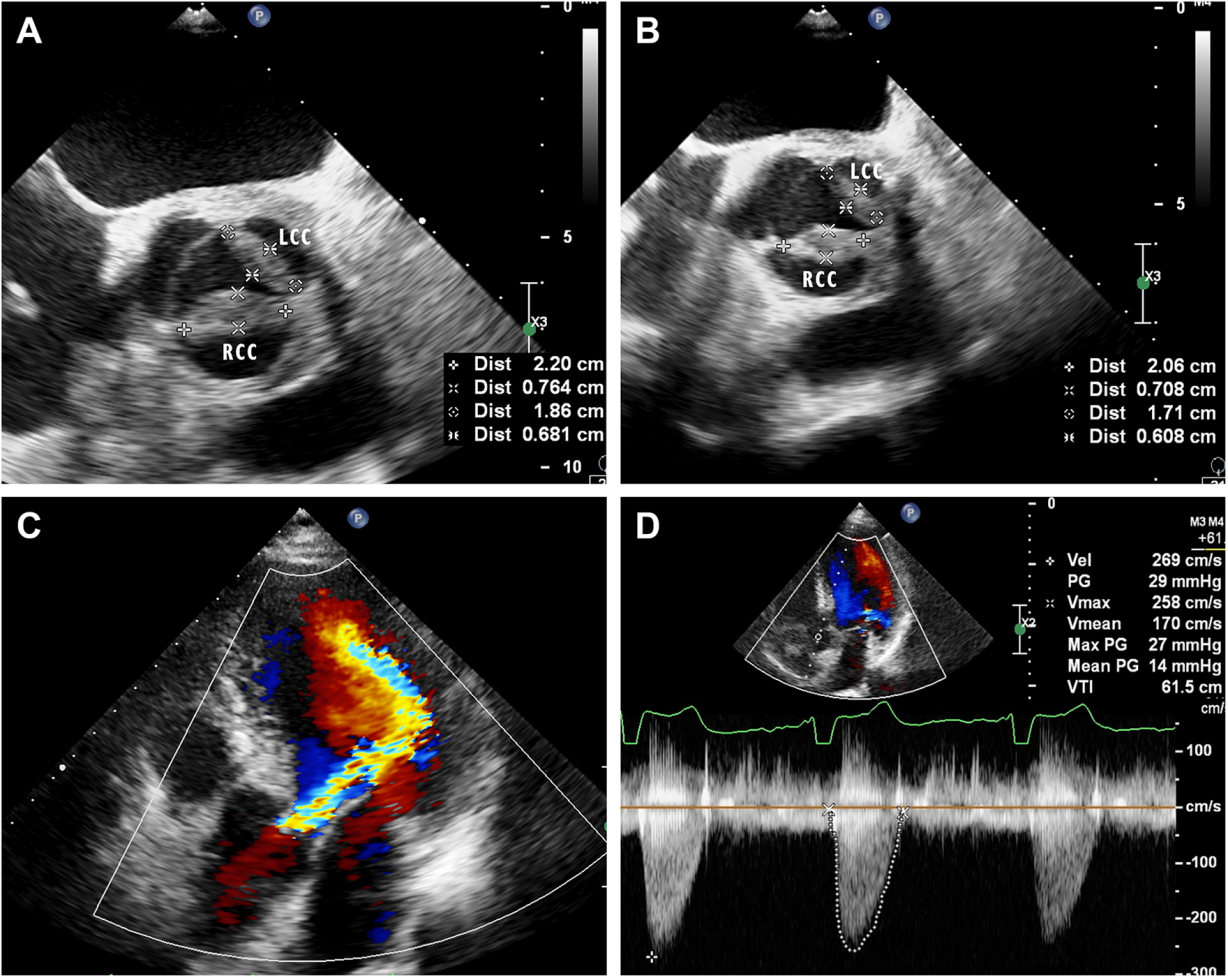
(A) Mid-esophageal aortic valve short-axis view of transesophageal echocardiography on admission showed that the left and right coronary valves of the aortic valve were 18.6 × 6.8 mm and 22.0 × 7.6 mm, respectively. (B) Mid-esophageal aortic valve short-axis view of transesophageal echocardiography after treatment showed improved thickening of the left and right coronary valves of the aortic valve, which were 17.1 × 6.1 mm and 20.9 × 7.1 mm, respectively. LCC, left coronary cusp; RCC, right coronary cusp. (C) Apical five chamber views of transthoracic echocardiography showed moderate regurgitation flow signal during diastole of the aortic valve. (D) Color Doppler ultrasound showed accelerated blood flow through the aortic valve during systole.
